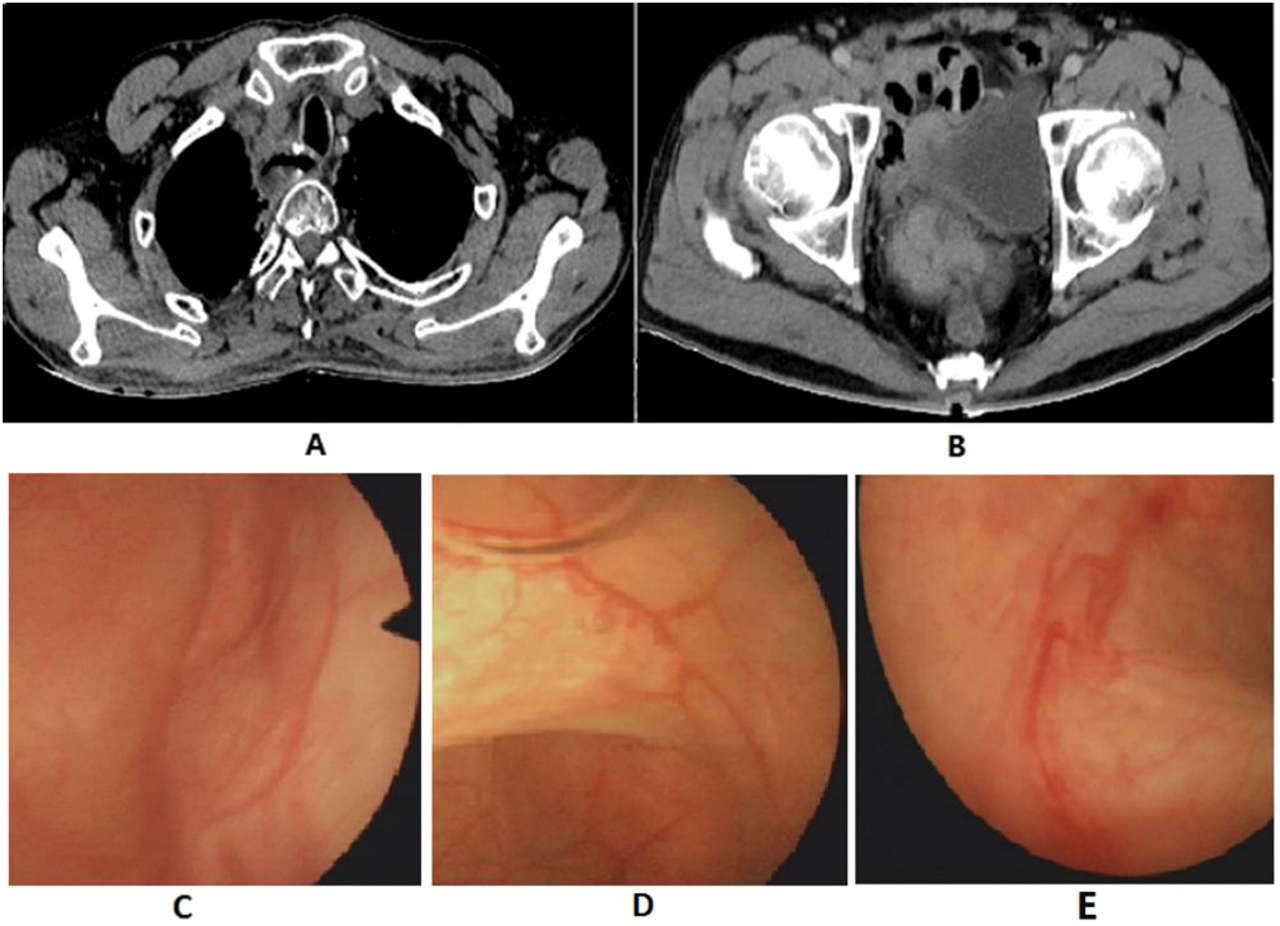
Postoperative follow-up imaging report. (A) Chest CT; (B) Pelvic CT; (C-E) Cystoscopy exam.
